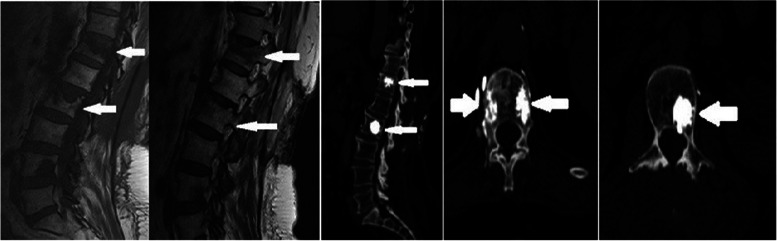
Sixty-four years old female patient. T12 and L2 vertebra metastatic involvement MRG images (preoperatively) and CT images after RFA and vertebroplasty
